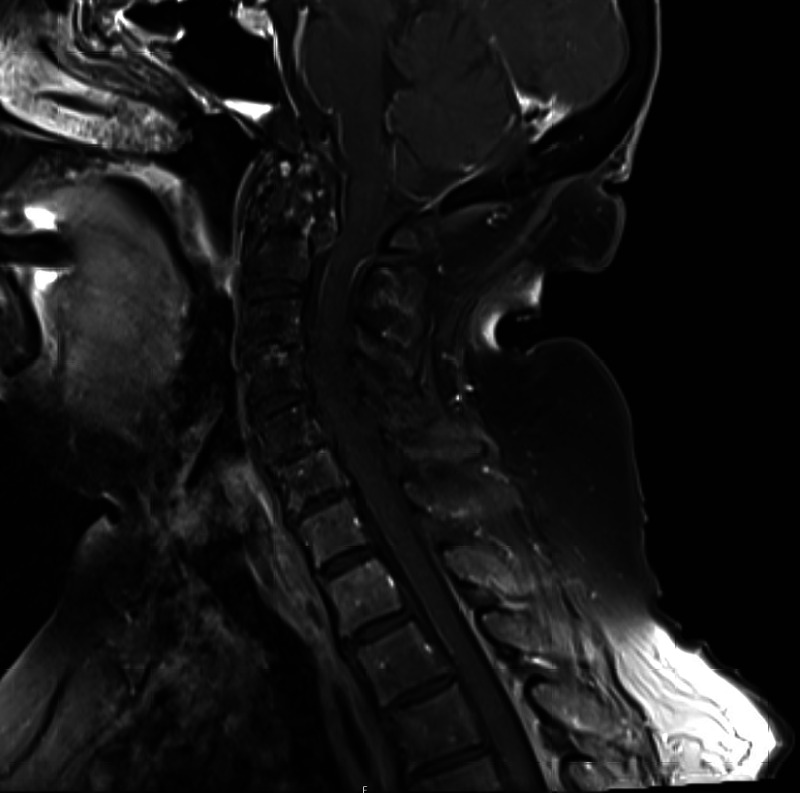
MRI cervical spine with and without contrast nine months later showing persistent meningeal enhancement. MRI, magnetic resonance imaging
